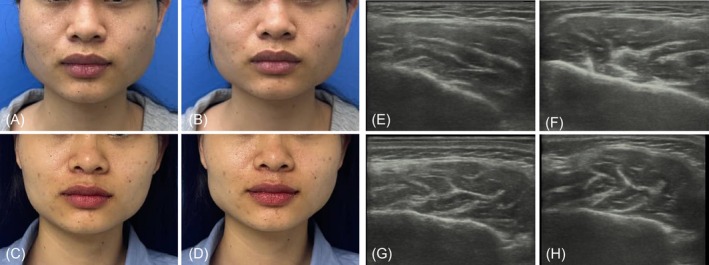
Case: A 27‐year‐old female. Preoperative photographs: (A) Resting state; (B) clenched contraction state. One‐month postoperative photographs: (C) Resting state; (D) clenched contraction state, showing abnormal bulging of the right masseter muscle. Pre‐injection B‐ultrasound images: (E) Resting state; (F) clenched contraction state. One‐month post‐injection B‐ultrasound images: (G) Resting state; (H) clenched contraction state, demonstrating abnormal contraction of the superficial masseter muscle.
